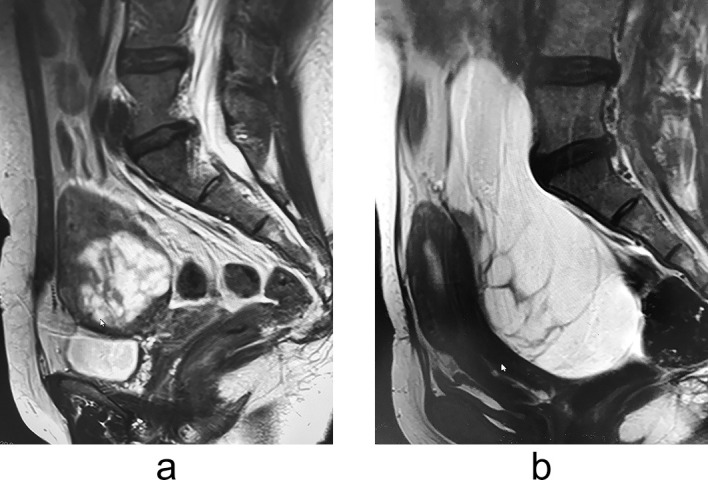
a MRI performed 9 months postoperatively showing a multilocular cystic lesion in the uterine cavity that was homogeneously hyperintense on T2-weighted images. b MRI performed 28 months postoperatively showing a 151-×61-×133-mm, well-defined, multilocular cystic mass on the exterior of the uterus. The lesion was homogeneously hyperintense on T2-weighted images.
